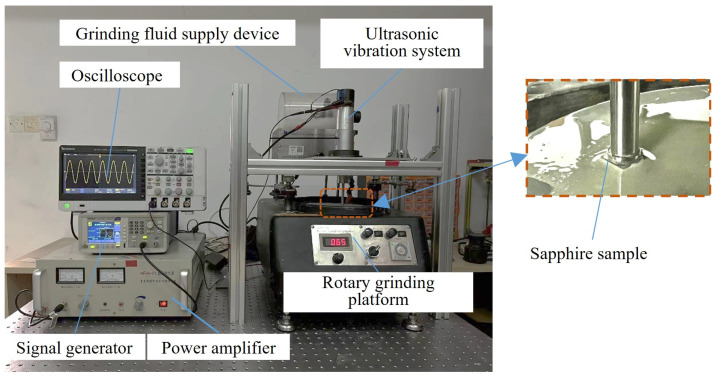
Experimental platform for ultrasonic grinding.
